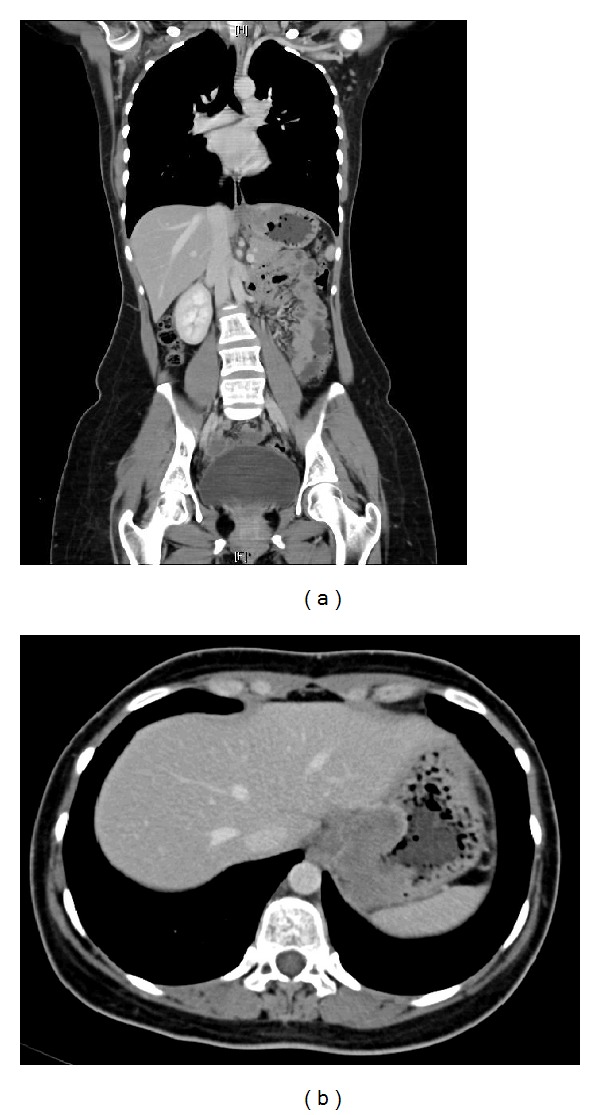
Coronal (a) and transverse (b) chest, abdominal, and pelvic contrast-enhanced computed tomography (CT) scans: showing a 3.8 × 7.2 × 8.7 cm ill-defined mass, involving gastric fundus and extending into gastric cardia and lower gastroesophageal junction. It was associated with multiple enlarged gastrohepatic lymph nodes. There was no evidence of ascites or retroperitoneal or mesenteric lymphatic metastases.
